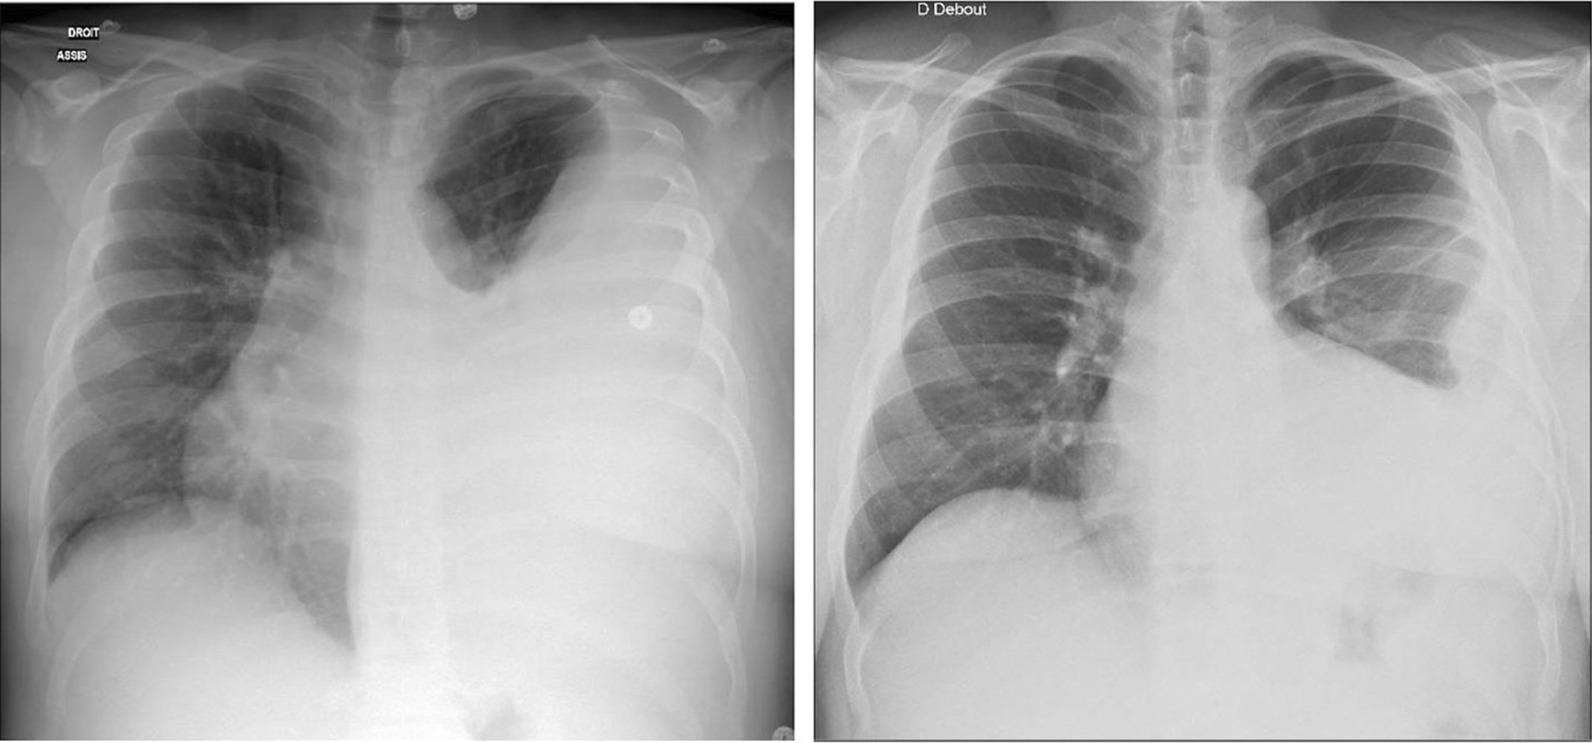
Chest x-ray shows left pleural effusion with superinfection before drainage (left) after drainage (right)
